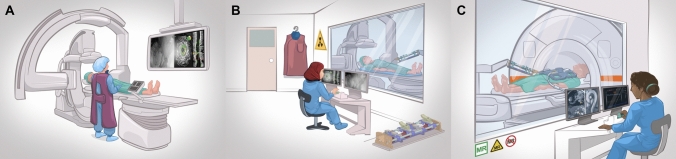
A Current, routine IR procedures during which the physician is exposed to ionising X-ray radiations, which may lead to a sizeable risk of cancer. The physician is also wearing a heavy lead apron, which makes the physician more prone to orthopaedic injuries. B Physician is remotely operating a robotic platform. The physician is no longer exposed to ionising radiations and no longer needs to wear the lead apron. The robotic platform may also improve the precision, stability, and comfort of endovascular procedures. C Physician is remotely operating a robotic platform inside an MRI scanner instead of using X-ray guidance. MRI does not have any ionising radiation, which makes it a safe option for patients, especially the paediatric population. MRI can also provide better visualisation of soft tissue and offers unparalleled 3D evaluations of pathology and function across the body
